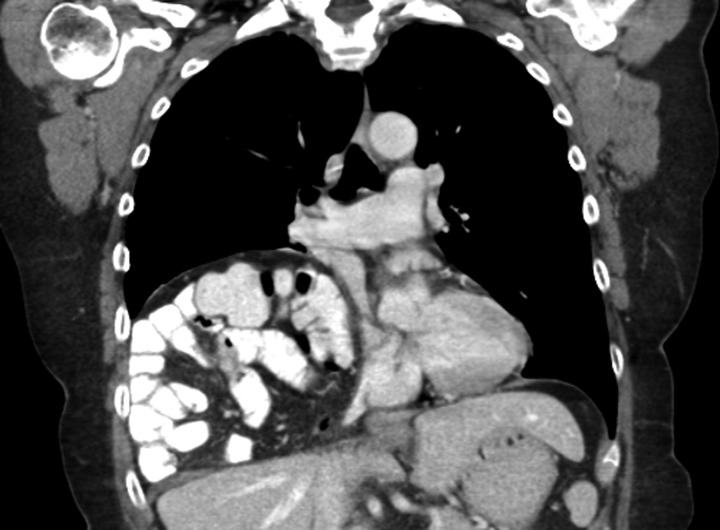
Another slice of the CT scan of the chest revealing the large size of this Morgagni hernia with multiple loops of small bowel and colon in the right inferior hemithorax.
